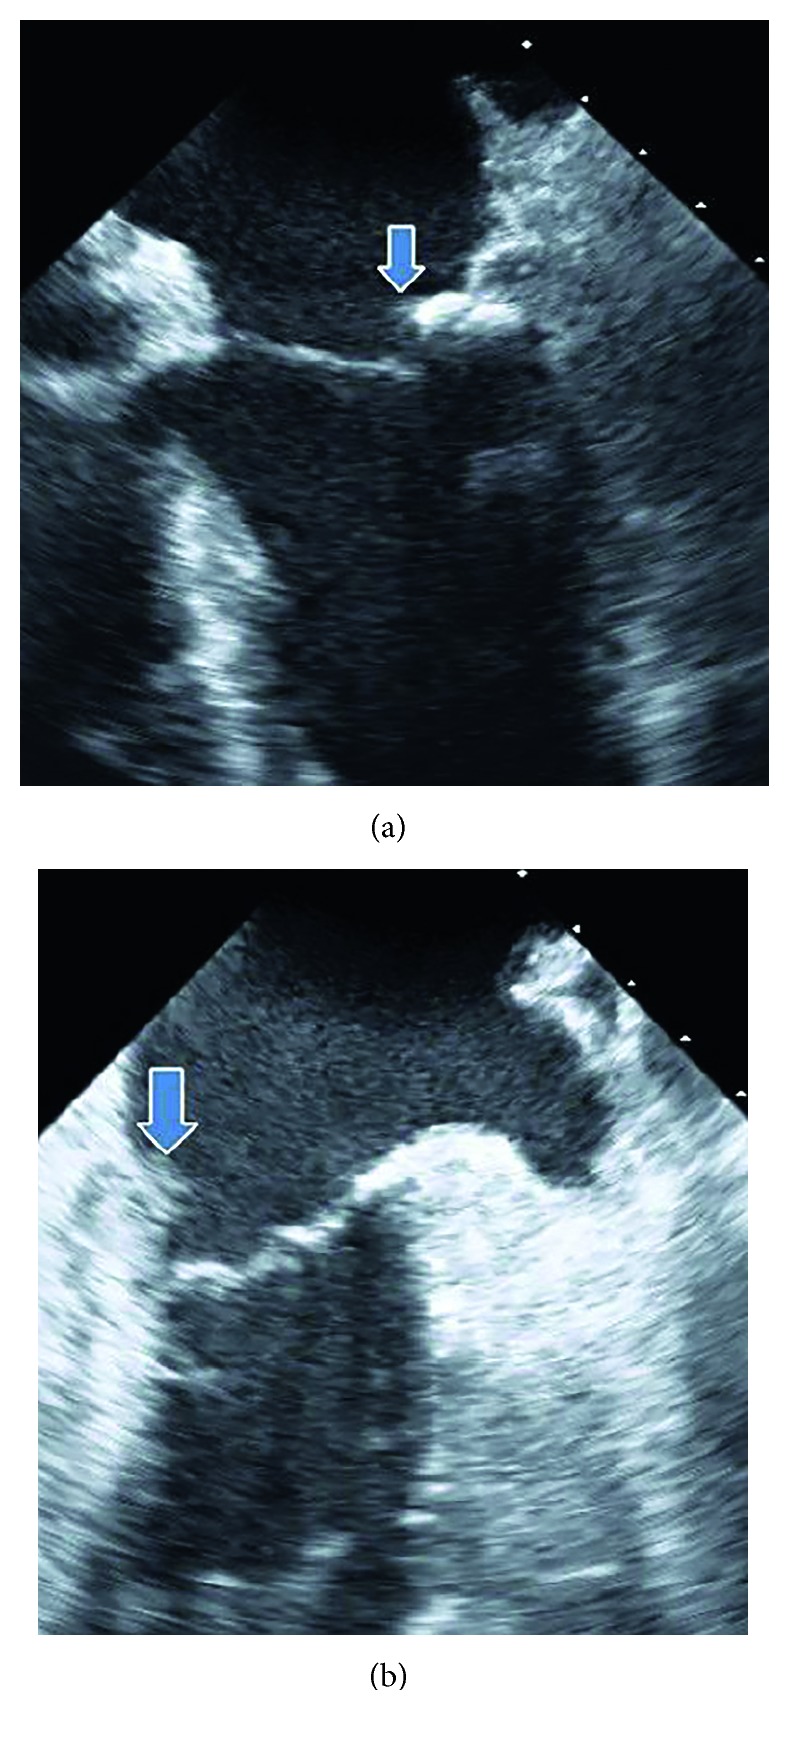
(a) Transesophageal echocardiography with a corner of 0°. Endocarditis vegetation of mitral valve is marked with arrow. (b) Transesophageal echocardiography with a corner of 90°. Endocarditis vegetation of mitral valve is marked with arrow.
